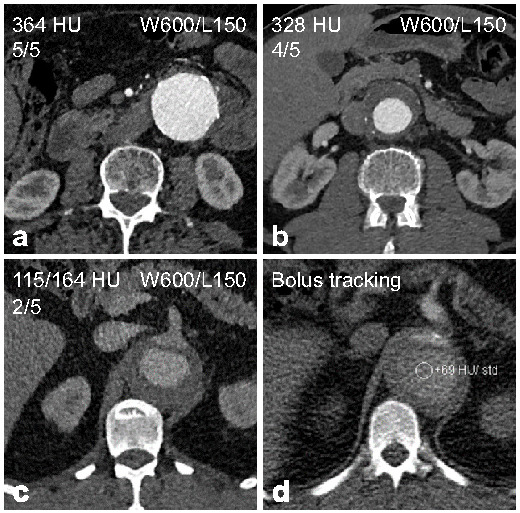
Representative examples for the low-dose CTA with identical window/level (W/L) settings. (a) CTA rated as excellent image quality. (b) CTA rated as good image quality. (c) CTA rated as fair image quality. (d) Bolus tracking of the same patient as in c showing the monitoring ROI placed in the false lumen of the aortic dissection.
